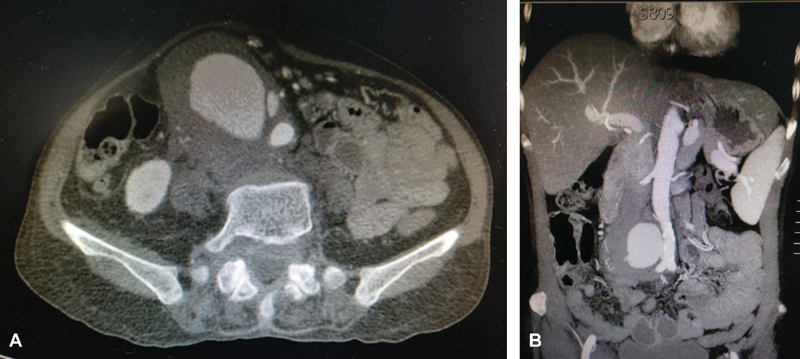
Computed tomography angiogram (axial [Panel A] and coronal [Panel B] views) showing saccular infrarenal aortic aneurysm with significant eccentric thrombus and focal dissection above aortic bifurcation.
